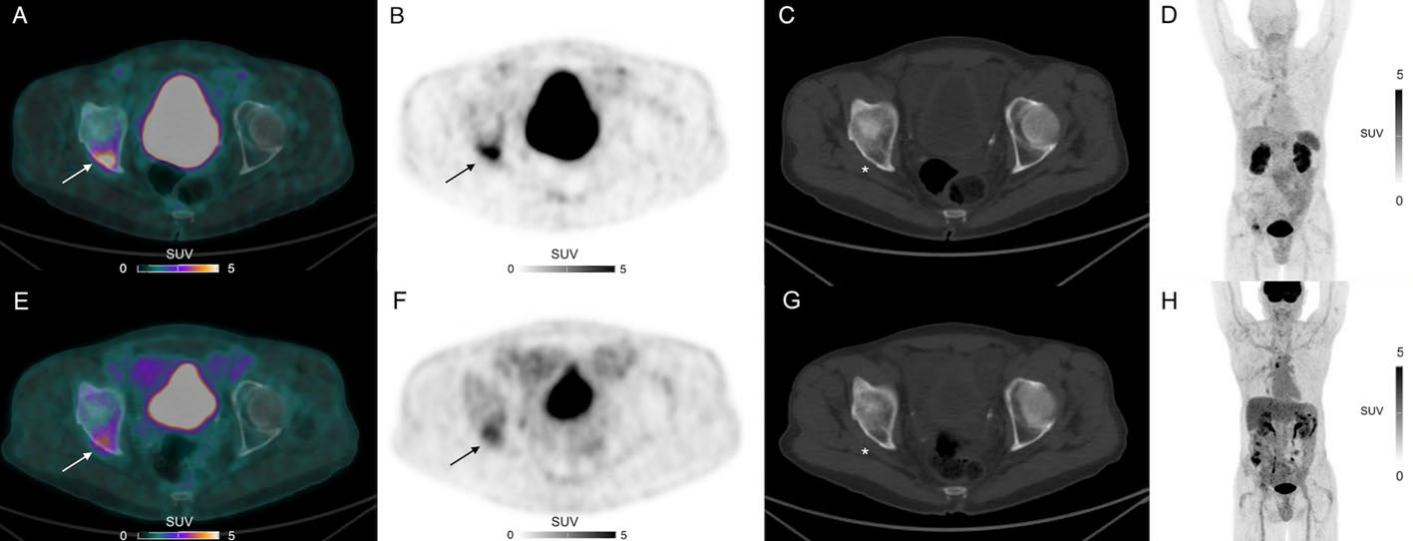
^68^Ga-NODAGA-RGD PET CT (A), PET (B, D) and CT (C) and ^18^F-FDG PET CT (E), PET (F, H) and CT (G) axial views showing more intense ^68^Ga-NODAGA-RGD uptake compared with ^18^F-FDG uptake (arrows) of an osteolytic lesion (asterisks) of a 63-year-old participant with a metastatic esophageal adenocarcinoma
